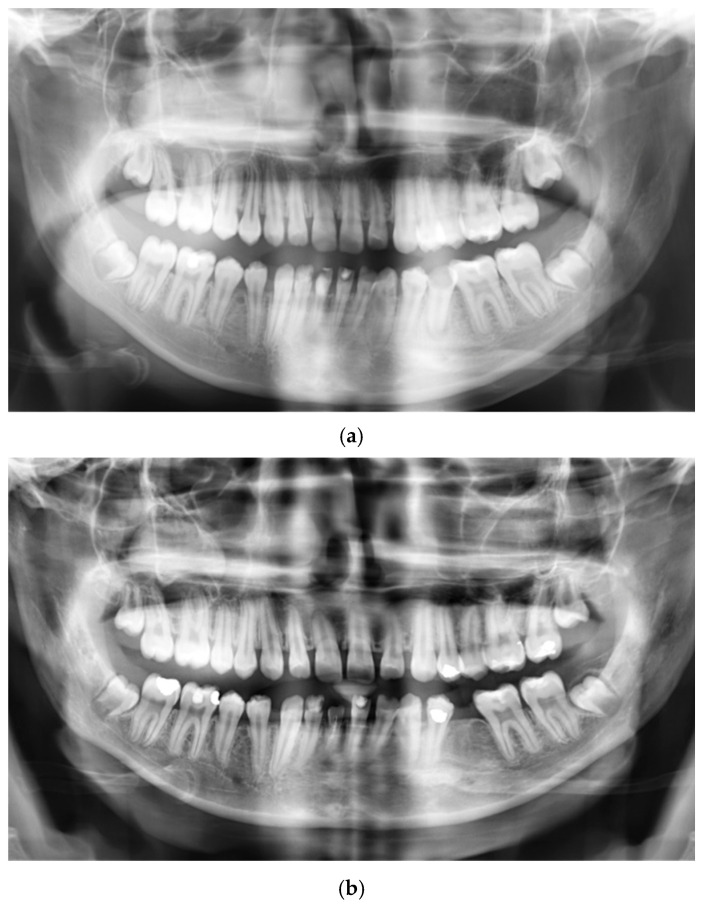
Patient n.1 pre- and post-treatment panoramic radiograph: (a) pre-treatment panoramic radiograph; (b) post-treatment panoramic radiograph shows conservative therapies, the root canal therapy of 4.1, the avulsion of 3.5 affected by periapical inflammatory disease.
